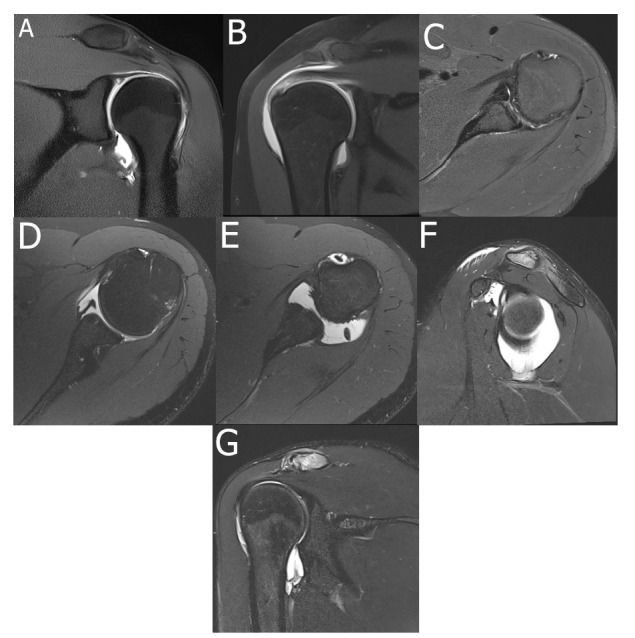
Magnetic resonance arthrography of the shoulder. (A) An MRA was performed on a 20-year-old male patient with shoulder instability and suspected capsular or labral structural damage after an inconclusive conventional shoulder MRI. Intra-articular gadolinium injection documents a deep sublabral fissure at the superior segment, compatible with a SLAP lesion on sagittal FS T2WI sequence. (B) Another patient who underwent MRA had a diagnosis of partial-thickness RC tear. However, after intra-articular gadolinium injection, there was filling of the subacromial-subdeltoid bursa, which was compatible with a full-thickness tear, as evidenced by coronal FS T1-weighted imaging (T1WI). (C) Conventional shoulder MRI in a 32-year-old patient with shoulder instability shows superimposing structures at the antero-inferior segment of the glenoid rim. Axial FS T2WI images reveal a bony Bankart with significant glenoid rim loss (D) and cortical stripping at the anterior-inferior segment (Perthes lesion) and a loose body (E) at the posterior recess. (A) FS T2WI sagittal (F) and coronal (G) MRA images of a 47-year-old patient with recurrent shoulder dislocation identify a capacious joint capsule with increased glenocapsular ratio and posterior laxity.
